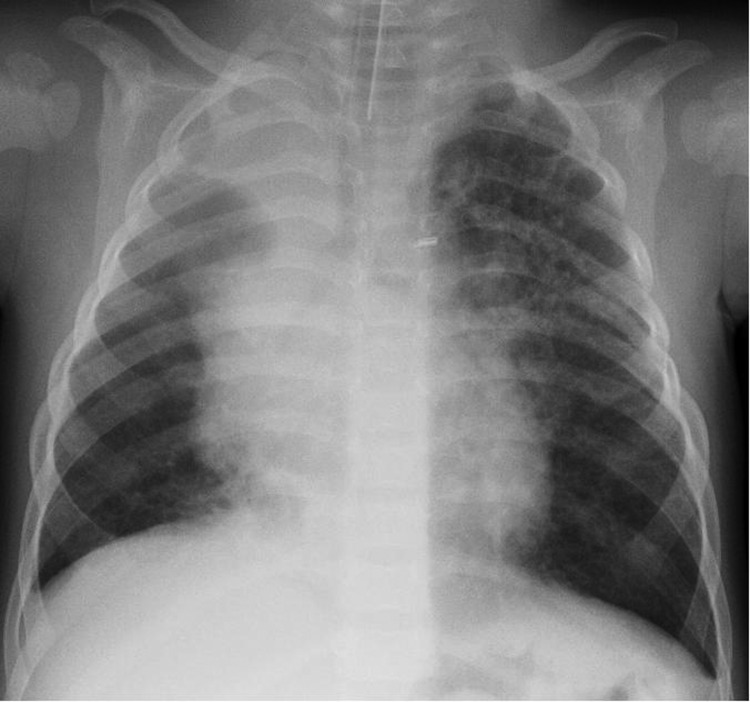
Chest radiograph of the index patient, a 16-month-old boy in Finland with human bocavirus 1 pneumonia, on day 2 of hospitalization. Bilateral pulmonary infiltrations and atelectasis of the upper right lobe can be seen.
